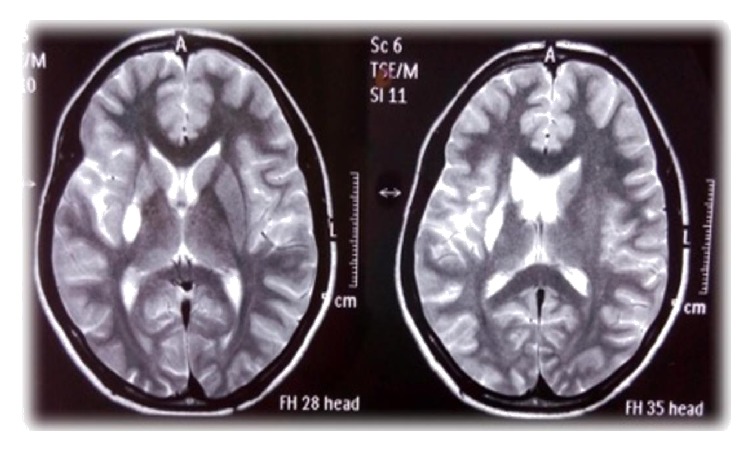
Axial T2-weighted MRI brain image showing infarct in right gangliocapsular region.
